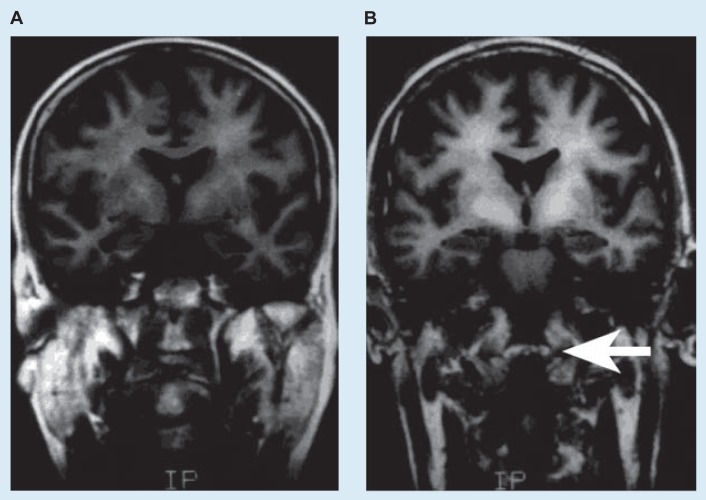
Magnetic resonance imaging of the pallidum in a healthy subject and in a patient with cirrhosis and portal-systemic encephalopathy. In the healthy subject (A), the pallidum has a normal appearance; in the patient (B), manganese accumulation produces an intense signal in the pallidum (indicated by arrow). (From Kulisevsky et al. 1992, with permission.)
